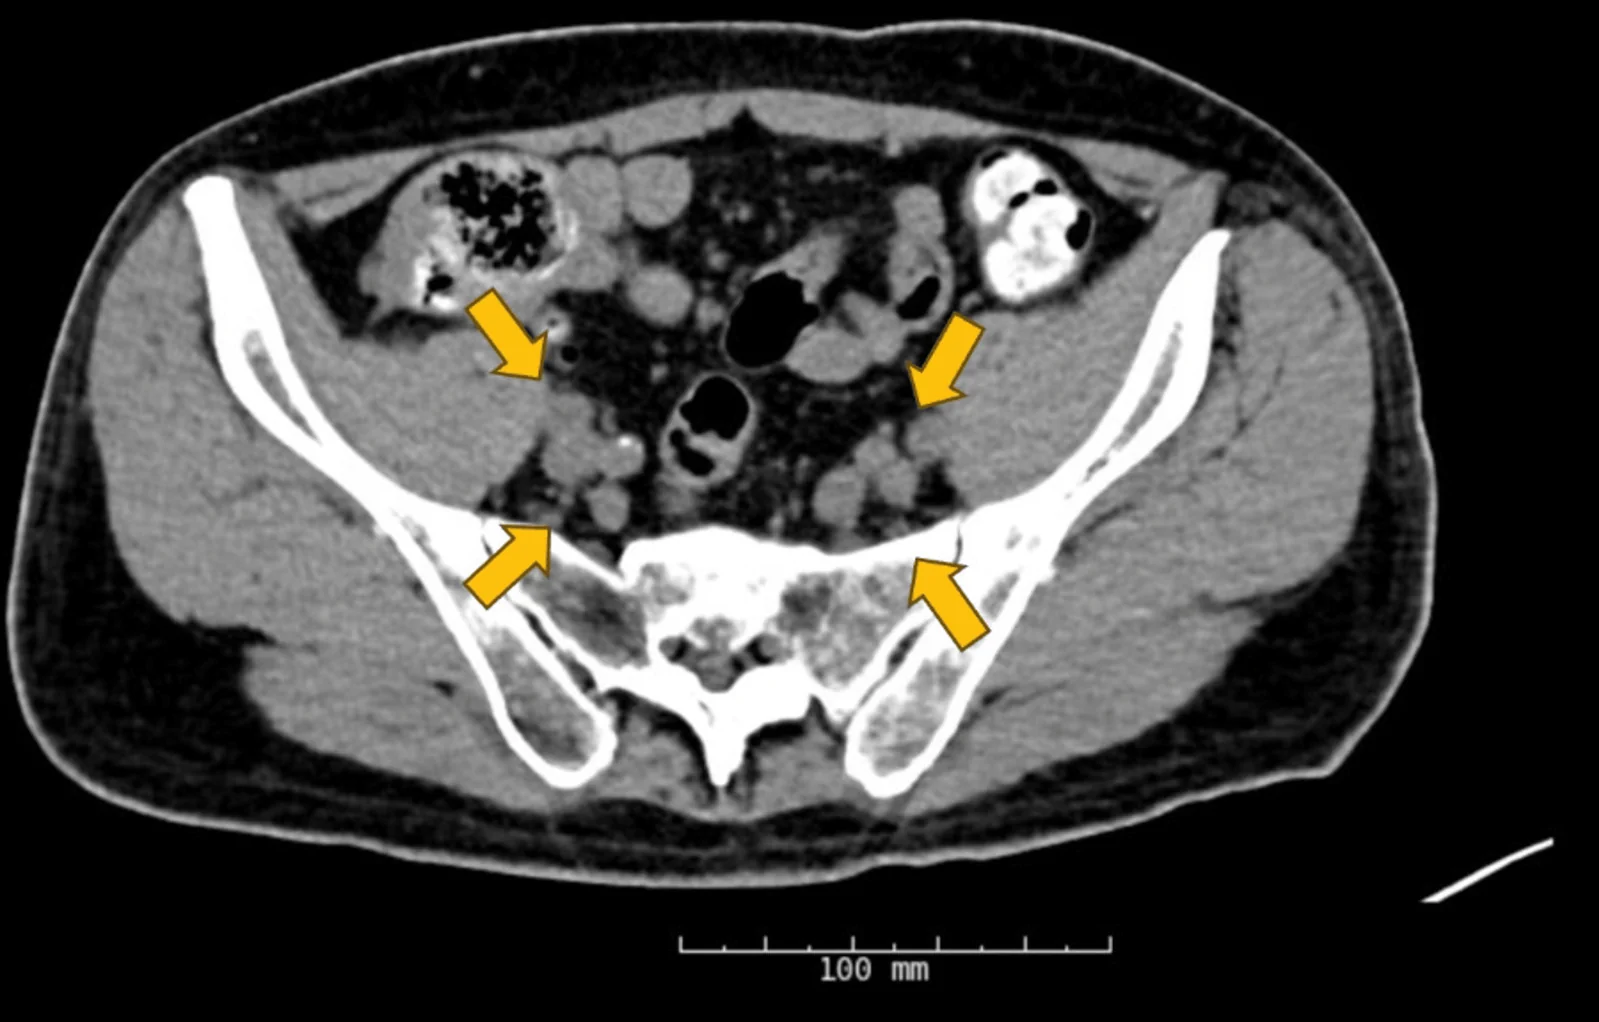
Preoperative abdominopelvic computed tomography of the recipient demonstrating suitable transplant vascular anatomy. Axial CT images of the recipient demonstrate the external iliac vascular regions evaluated preoperatively for transplant suitability, indicated by yellow arrows. No anatomic vascular contraindications to transplantation were identified.
